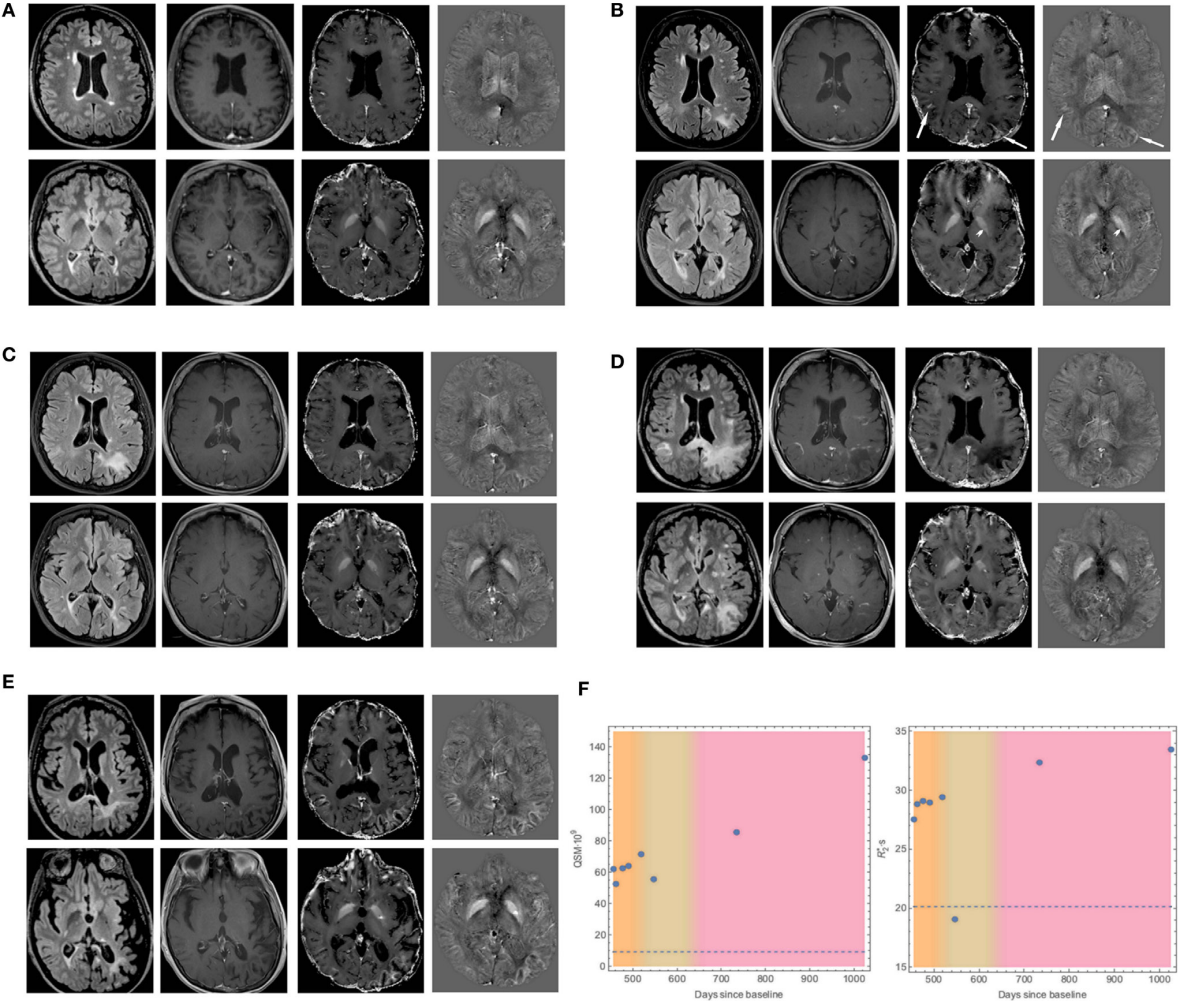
Evolution of MRI findings in our case of natalizumab-related progressive multifocal leukoencephalopathy (PML). Axial fluid-attenuated inversion recovery (FLAIR, first column), contrast-enhanced T1-weighted images (second column), R2* maps (third column), and quantitative susceptibility maps (QSM, fourth column), of two levels (ventricular bodies and basal ganglia planes) from brain MRI scans performed in a phase of multiple sclerosis stability (A), in the early symptomatic PML phase (B), at a PML follow-up (C), when Immune Reconstitution Inflammatory Syndrome (IRIS)-PML ensued (D), and at a chronic stage (E). (A) Multiple periventricular and juxtacortical FLAIR-hyperintense demyelinating lesions, non-enhancing after i.v. gadolinium administration, and without significant susceptibility modifications on R2* or QSM maps. (B) Evidence of new FLAIR-hyperintense bilateral parieto-occipital subcortical lesions, with mild contrast enhancement and clearly increased values of R2* and QSM in the adjacent U-fibers (arrows). Furthermore, a small non-enhancing, FLAIR-hyperintense lesion in the left globus pallidus is present, showing mildly decreased values on R2* and QSM maps (arrowheads). (C) Progressive extension of the previously described lesions showing lack of significant contrast-enhancement and constantly high R2* and QSM values in adjacent U-fibers. The left pallidal lesion now shows a mild increase in susceptibility on R2* and QSM maps. (D) Further extension of FLAIR-hyperintensities with most of the lesions vividly enhancing after contrast administration. U-fibers high values on R2* and QSM maps are still present, whereas the susceptibility changes in the left globus pallidus become more evident. (E) Reduction of the lesion load with significantly increased values on R2* and QSM maps of adjacent U-fibers, associated with brain atrophy. The left globus pallidus’ lesion shows a punctate hypointense signal on FLAIR images, corresponding to a significant increase of R2* and QSM values. (F) Plots showing the time evolution of QSM and R2* values in the U-fibers adjacent to the left parieto-occipital subcortical lesion, with reference to the baseline (dotted line). The three colored bands represent different phases of the disease (PML, PML–IRIS, and chronic stage).
